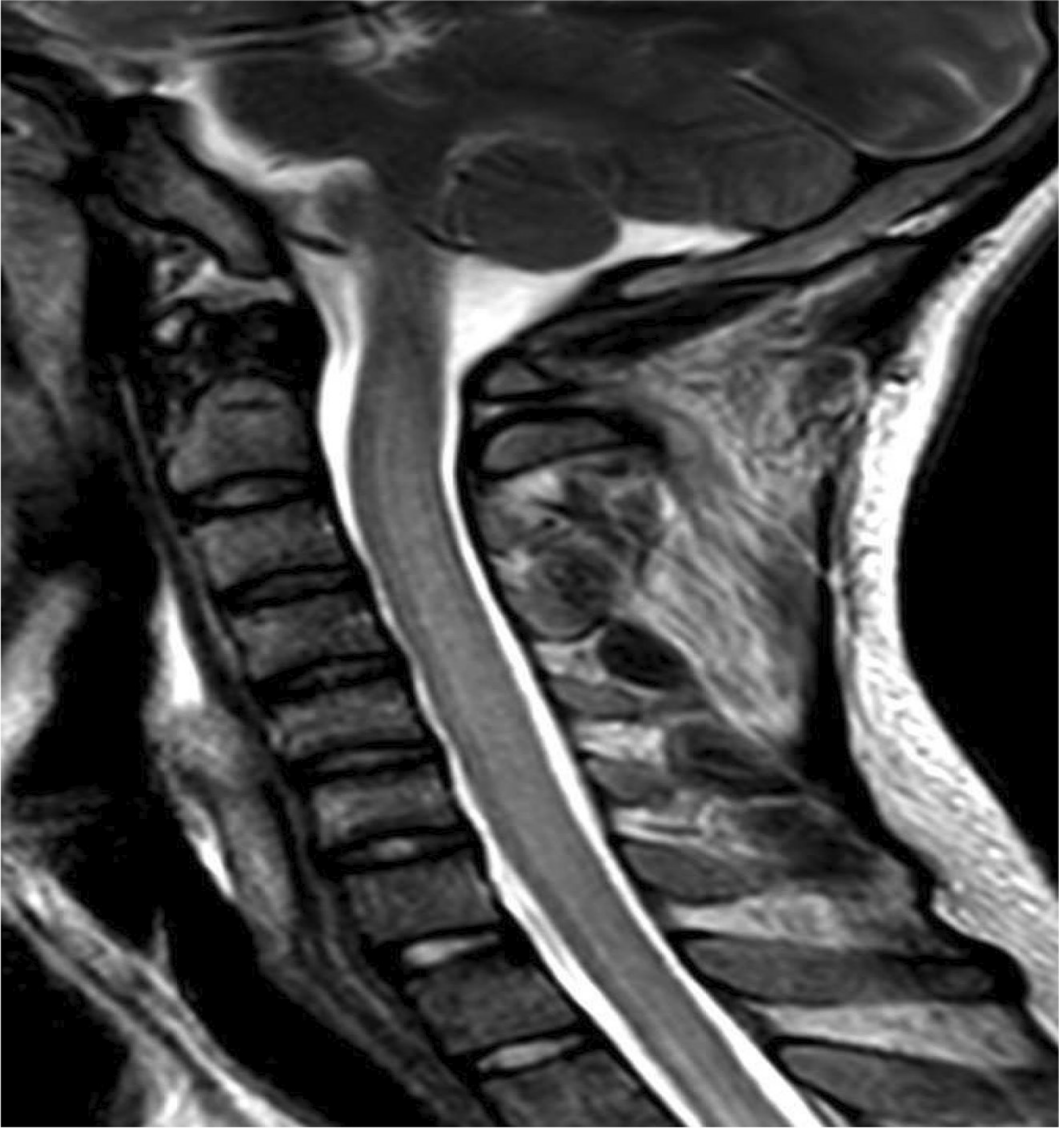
MRI Spine T2 Sagittal: showing cervical cord edema.
